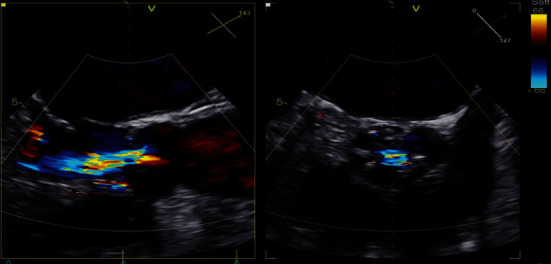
Transesophageal echocardiography image acquired prior to TAVR demonstrating thickened aortic valve with significant central aortic regurgitation without evidence of vegetation or perforation.
